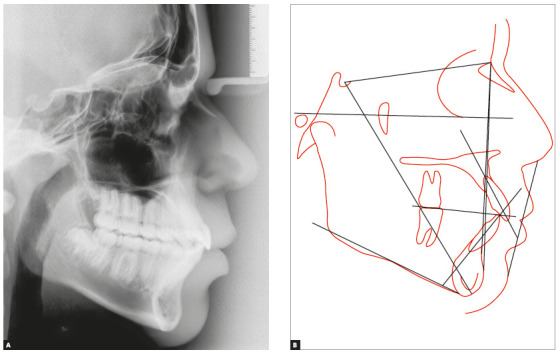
Final cephalometric radiograph (A) and final cephalometric tracing (B).
